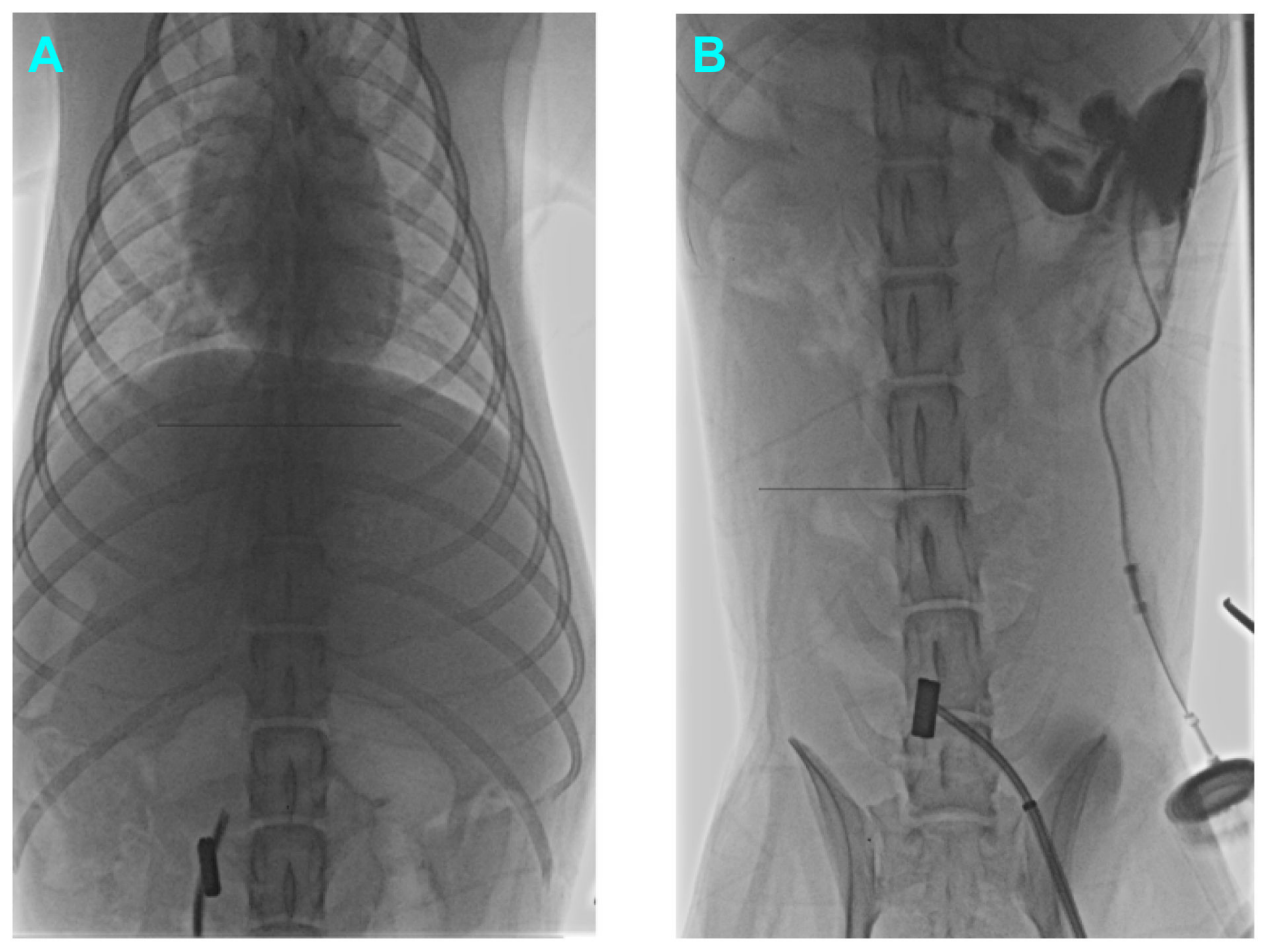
Procedure of the second surgery. A, Under X-ray guidance, the needle of the rosch-uchida transjugular liver access set was advanced slowly and ceaselessly along the outline of the magnets. B, The magnets were pulled out of the body by the wire fixed to the parent magnet (X-ray photograph).
